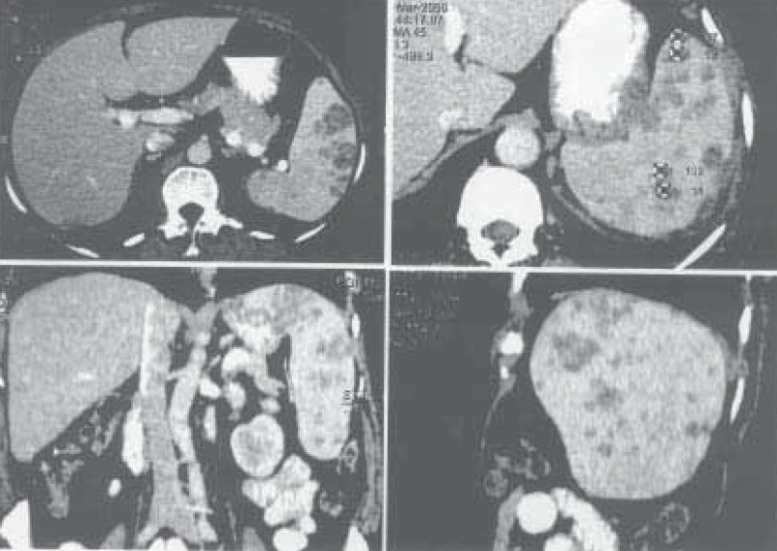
Computed tomogram of abdomen (A normal view, B enlarged view, C coronal section, and D sagital section) showing multiple small focal hypodense lesions that were suggestive of abscesses.
